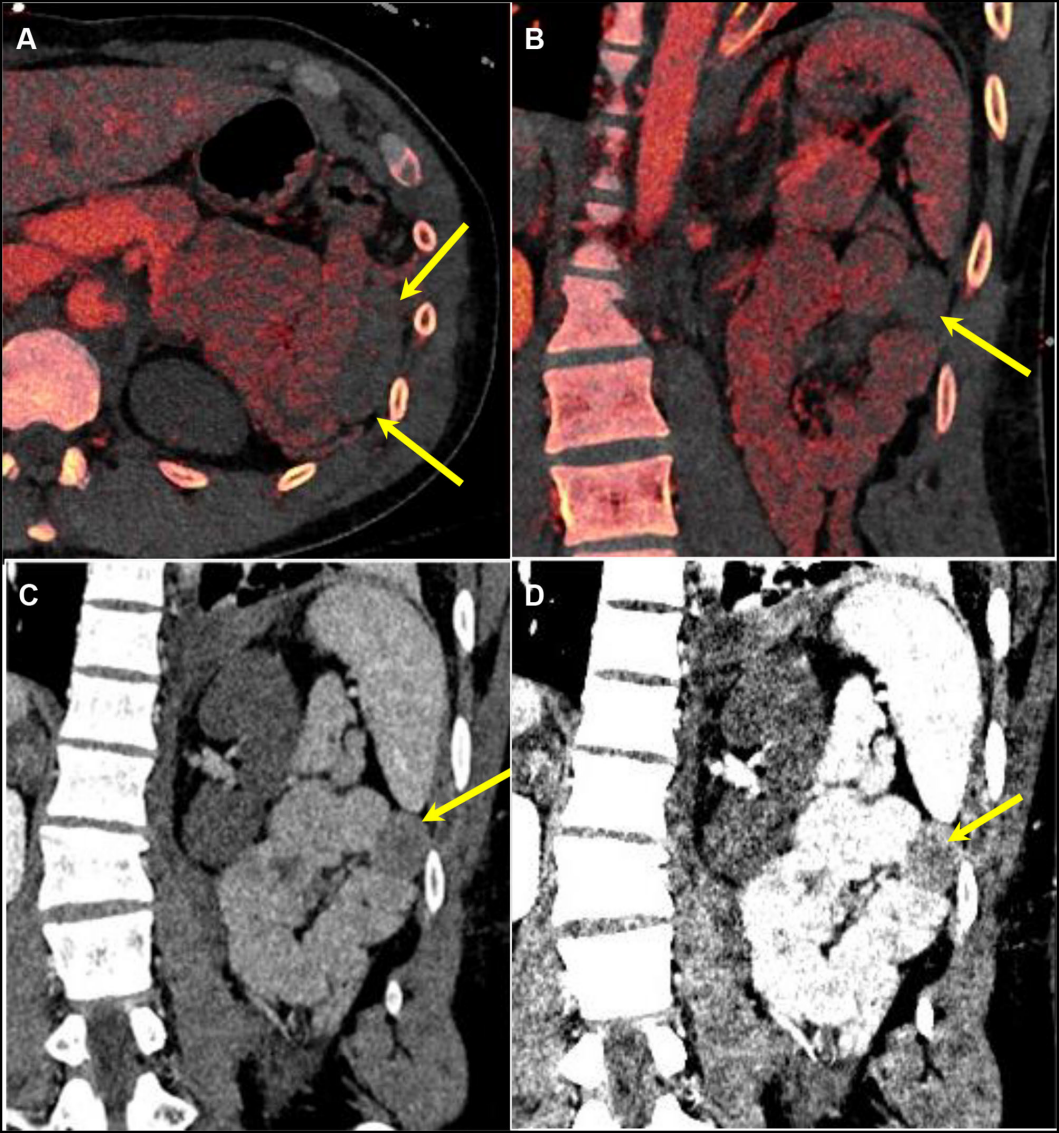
A 25-year-old male patient presenting after a high-speed motor vehicle accident. [(A) Axial and (B)] Coronal DECT iodine overlay images demonstrating decreased iodine uptake in a left upper quadrant small bowel loop compared to the adjacent loop (yellow arrows). DECT VMI reconstructions at (C) 70 keV (equivalent to a conventional CT at 120 kVp) and (D) 40 keV demonstrating a higher difference in attenuation between the normal and abnormal loops at a lower energy level. DECT, dual-energy CT; VMI, virtual monoenergetic images.
